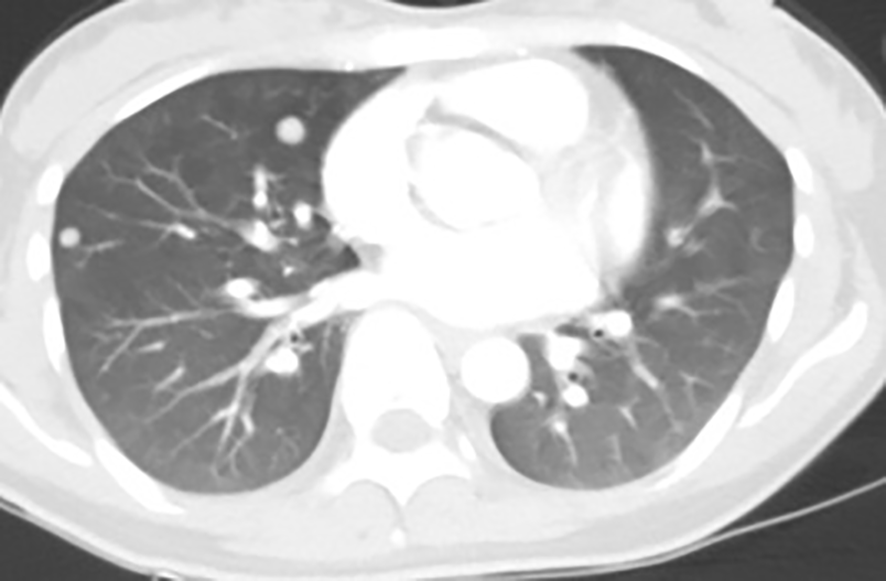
CT scan of the chest showed numbers of nodules with tissue density distributed on both lungs.
